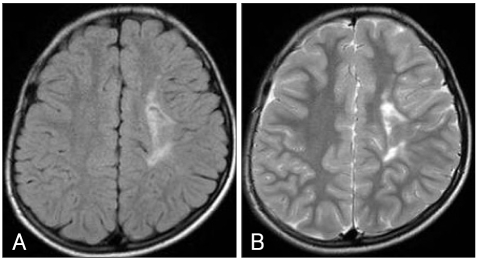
FLAIR and T2-weighted brain MR image. A and B show a high signal intensity lesion on FLAIR and T2-weighted brain MR image, highly suggestive of periventricular leukomalacia, in the left corona radiata and centrum semiovale (Taken when the patient was 10 years old). FLAIR: fluid attenuated inversion recovery.
